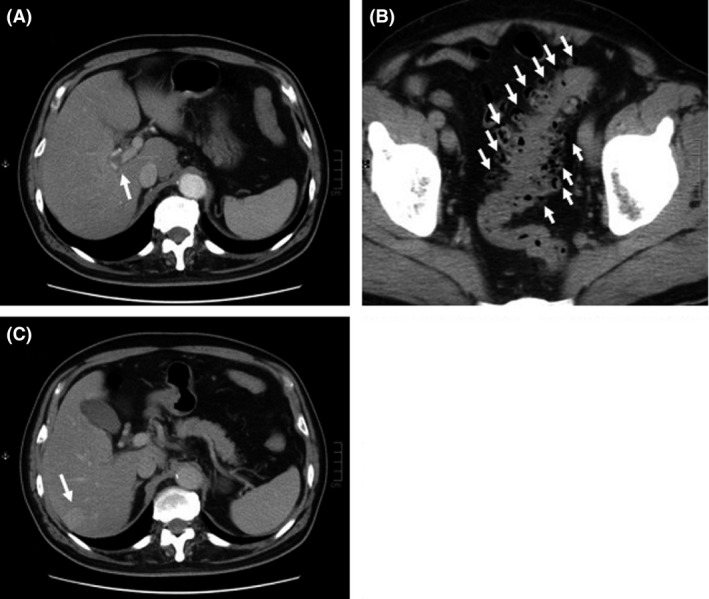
Contrast‐enhanced computed tomography images of the abdomen on admission. (A) Intravascular thrombosis was observed in the right portal branch (white arrow). (B) Multiple diverticulae were also observed in the sigmoid colon (white arrows). (C) An approximately 2‐cm‐diameter lesion was observed in S7; this was slightly enhanced in the equilibrium phase.
